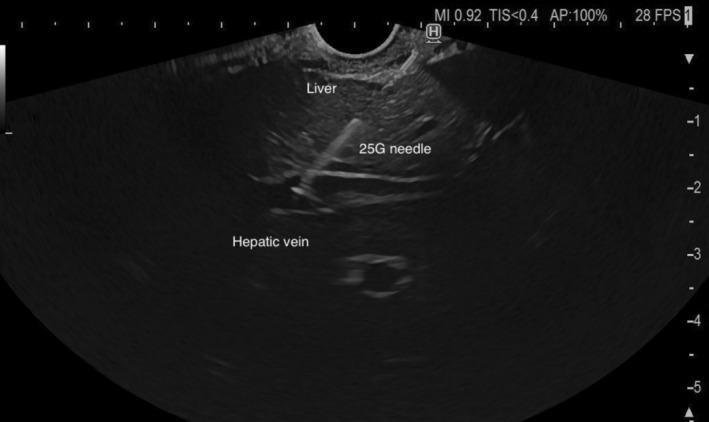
Endoscopic ultrasound‐guided measurement of hepatic vein pressure.
